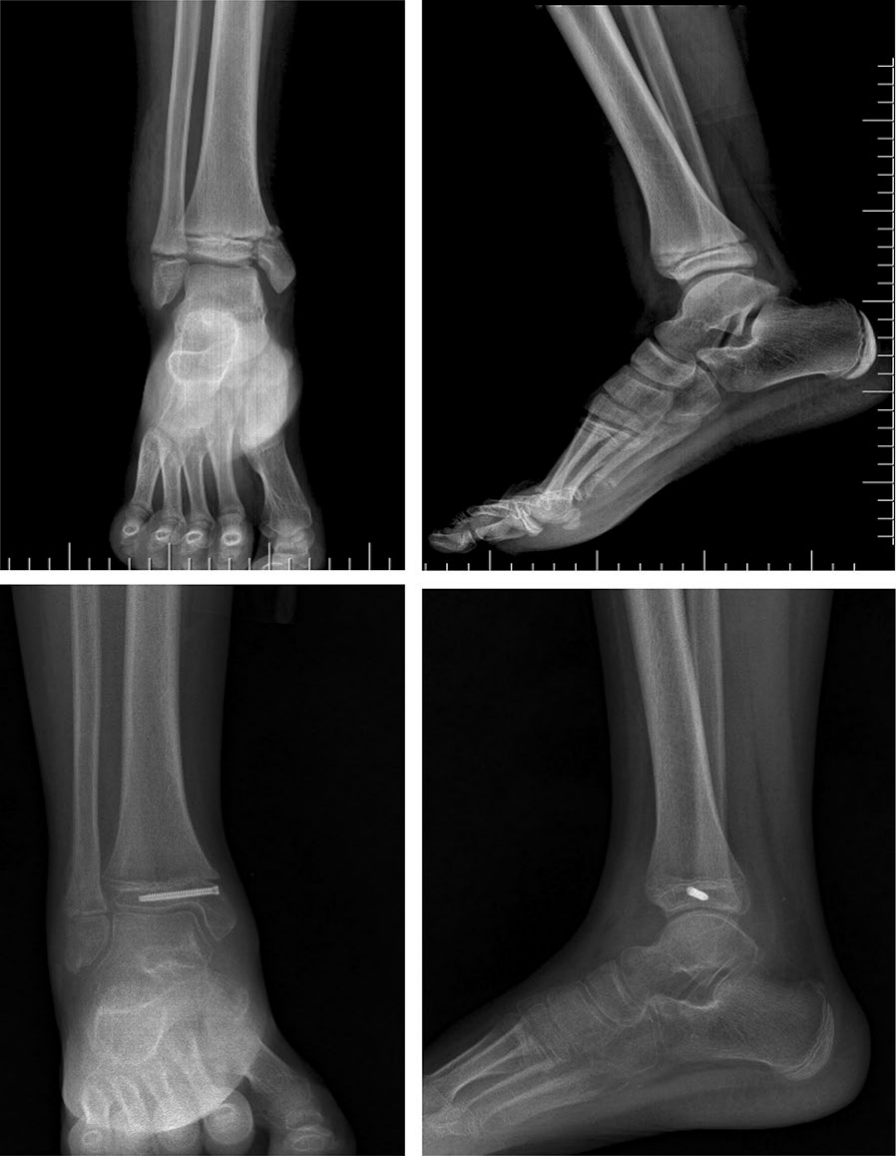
A 12-year-old boy with Salter-Harris type 4 physeal fracture. Pre-operative and post-operative X-rays after 3 months
